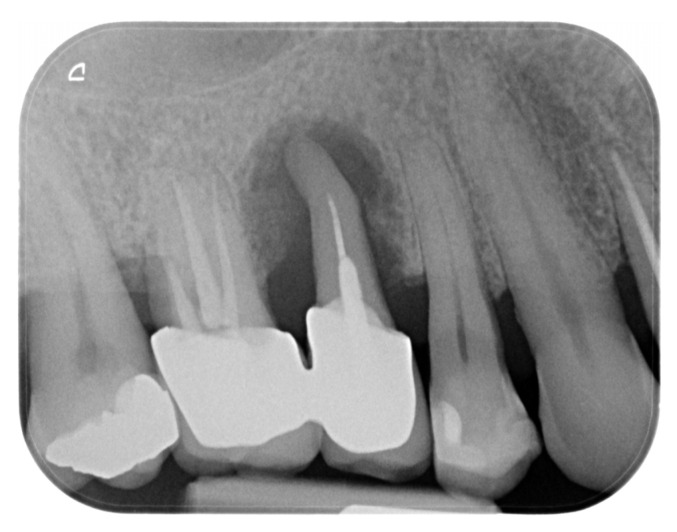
Initial X-ray status prior to clinical examination.
